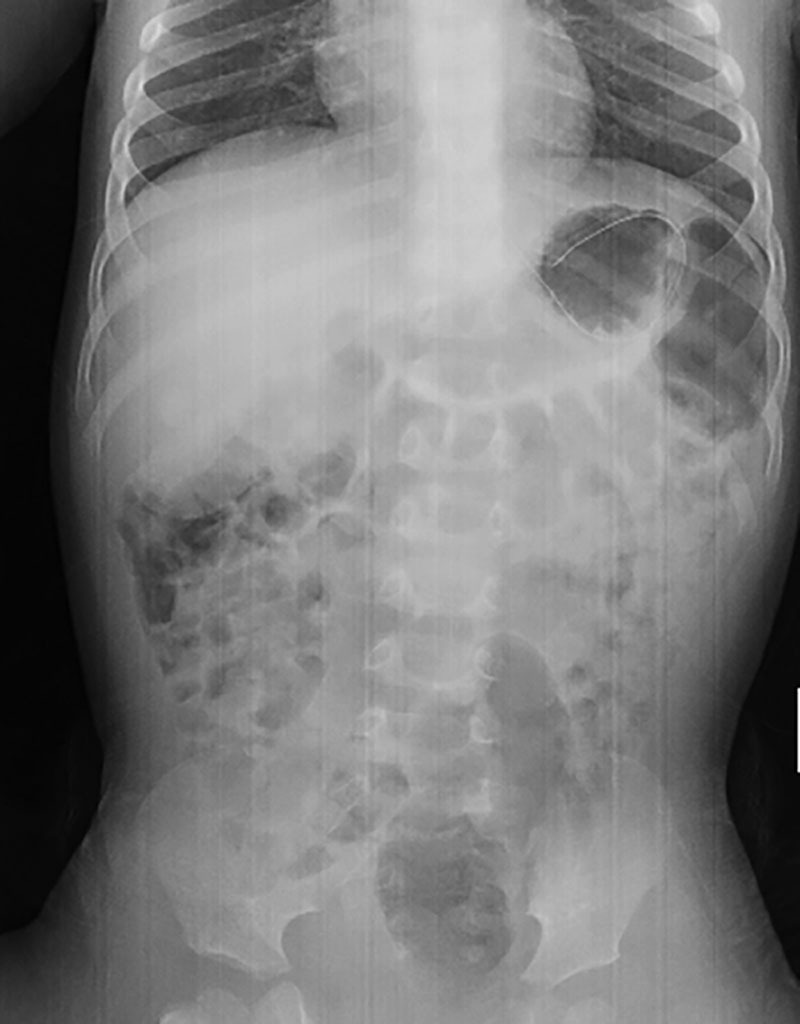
Plain abdominal radiograph with nonobstructive bowel gas pattern, demonstrating appropriate position of nasogastric tube.
